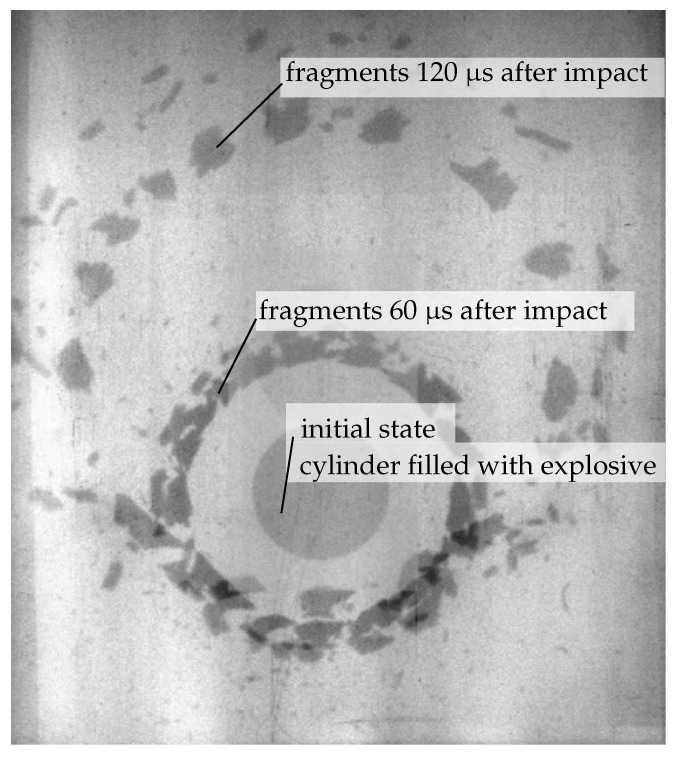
Double-exposed X-ray visualization of ring expansion for 1:2 aspect ratio (exposures 60 μs and 120 μs after detonation).
